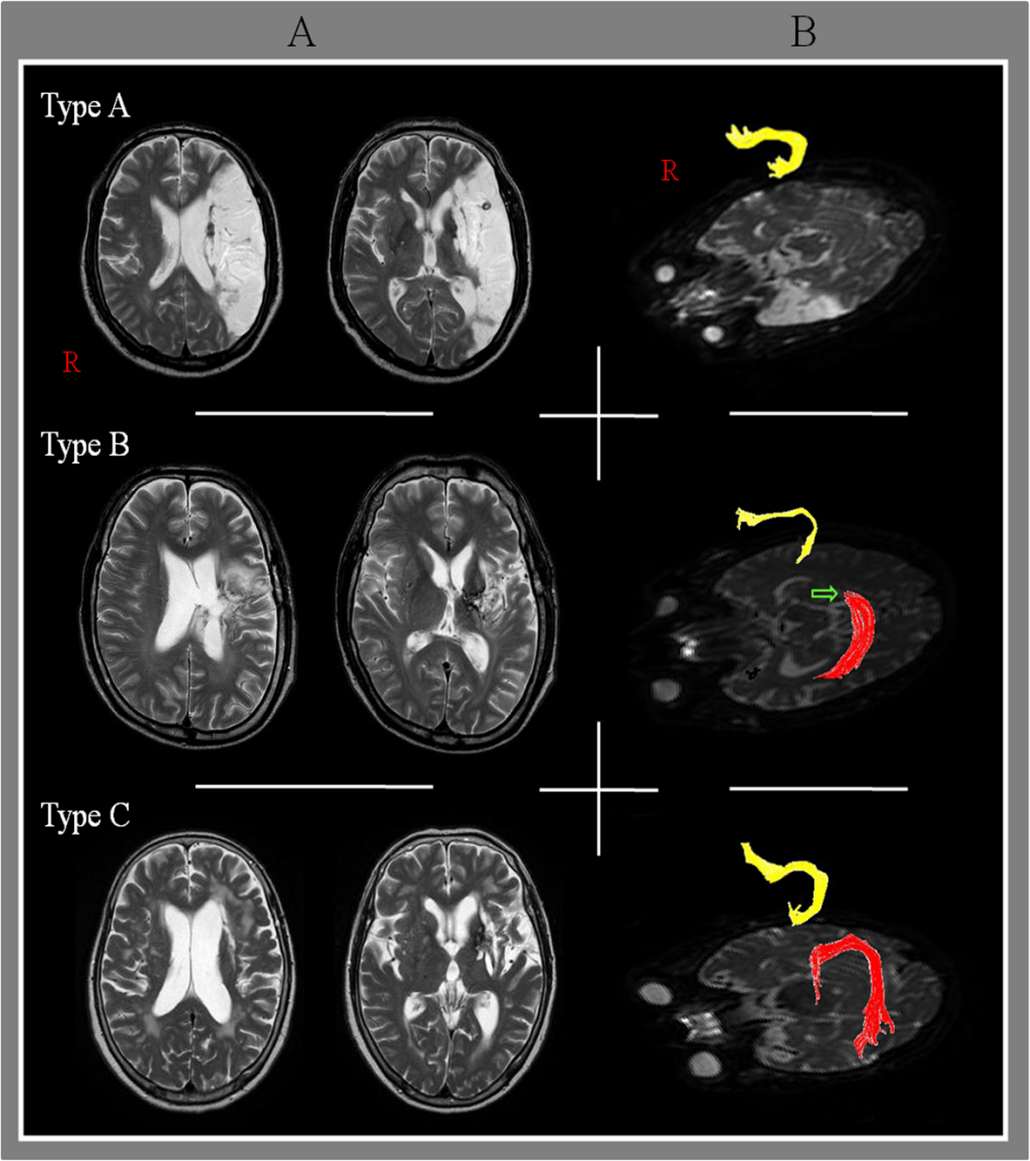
Classification of aphasia patients according to severity of the arcuate fasciculus impairment. (A) T2-weighted brain MRI in chronic stroke patients with aphasia, (B) diffusion tensor imaging tractogrophy for the arcuate fasciculus (AF) (Right AF: yellow color, left AF: red color, arrow: discontinuation).
